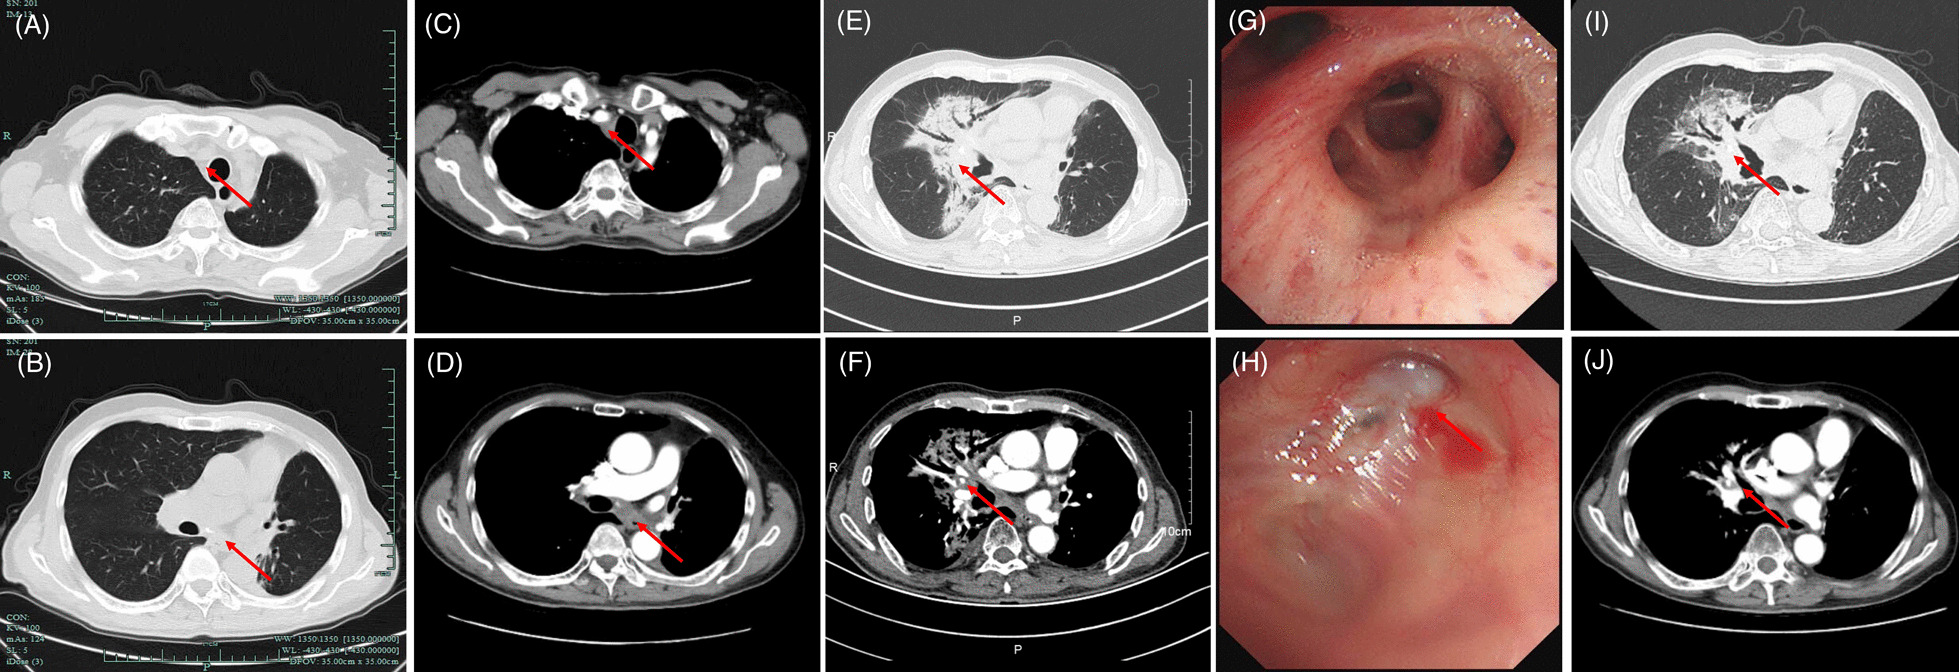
CT lung window (A, B) and contrast window (C, D) before pembrolizumab plus nab-paclitaxel, captured on November 5th, 2020; E, F CT lung and contrast window on May 1st, 2021; G The mucosa of right middle bronchus: is normal and the bronchus is clear. H The mucosa of the left upper lobe is post-surgery appearance and the bronchus is clear. I, J CT lung and contrast window captured on June 5th, 2021 (one month after steroids). Significant abnormal findings noted (arrow)
